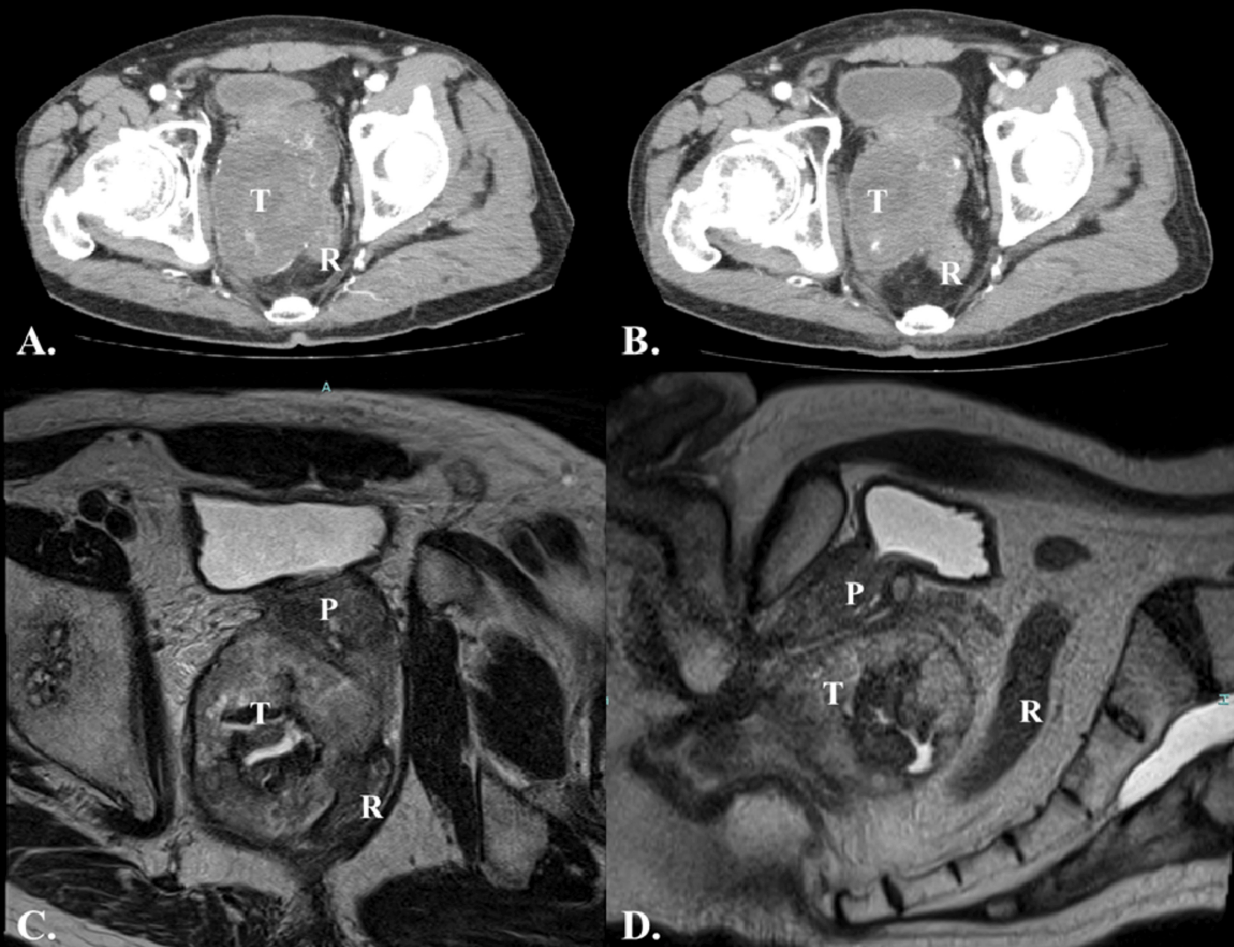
Pretreatment computed tomography (CT) images and post-neoadjuvant imatinib treatment images (A) Pretreatment contrast-enhanced CT shows the tumor (128 mm in diameter) is present in the right anterolateral wall of the rectum. (B) Contrast-enhanced CT shows tumor shrinkage (80 mm in diameter). (C,D) Magnetic resonance imaging shows rectal compression by the tumor and contact with the prostate, with a slight border between the tumor and the prostate. (B-D) Post-neoadjuvant imatinib treatment images. P, prostate; R, rectum; T, tumor
